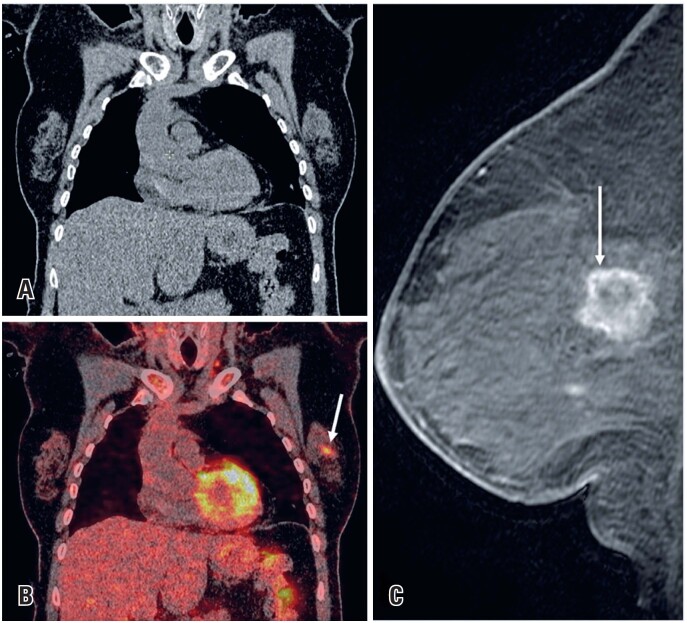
A 65-year-old woman with Lynch syndrome type II was being investigated for an ovarian neoplasm. (A and B) A PET-CT scan identified a hypermetabolic area in the left breast (arrow). A core biopsy confirmed invasive ductal carcinoma. (C) A subsequent breast MRI sagittal T1 - weighted contrast-enhanced imaging showed an irregular breast mass with rim enhancement and washout (arrow)
